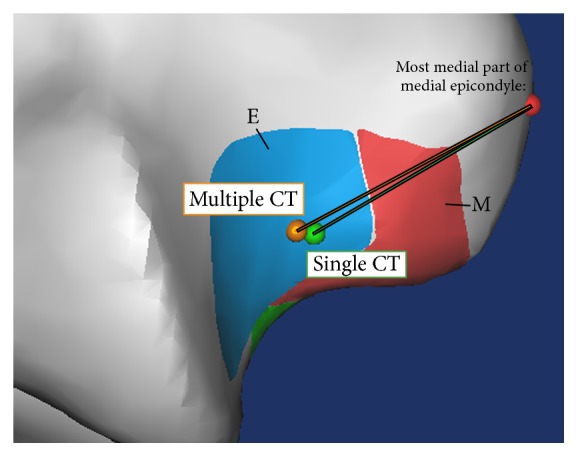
Isometric ligament origin locations relative to the medial epicondyle (red ball) after being analyzed with single CT (green ball) and multiple CT scan methods (orange ball).
